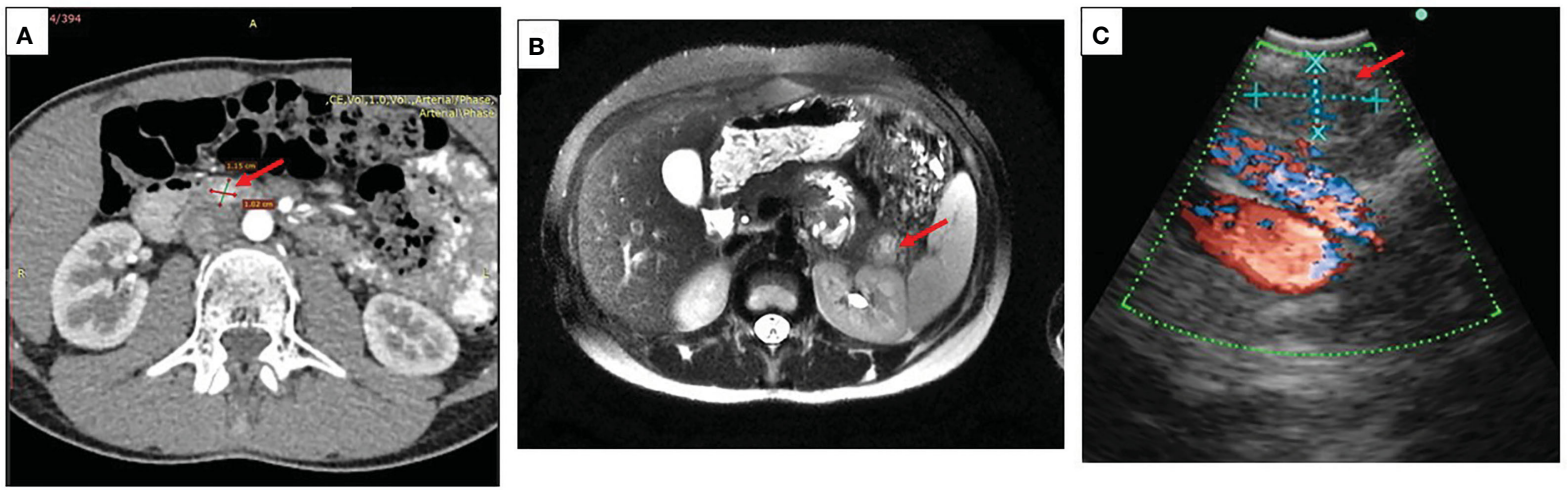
Imaging in pediatric patients with insulinoma (A) A representative CT scan showing insulinoma in the head of the pancreas (case #14), arrow indicating a round shaped hypervascular 1.5 cm lesion in the head of the pancreas highly enhanced during the arterial phase; (B) A representative contrast MRI scan showing an insulinoma in the pancreatic tail (case #8), arrow indicating a 2.3 cm lesion in the pancreatic tail with high signal intensity on T2 MR regimen; (C) A representative picture of endoscopic ultrasound localizing lesion in the pancreatic tail (case #13), arrow indicating a 0.9 cm ovoid shaped lesion, isoechoic with hypoechoic inclusions and distinct margins.
